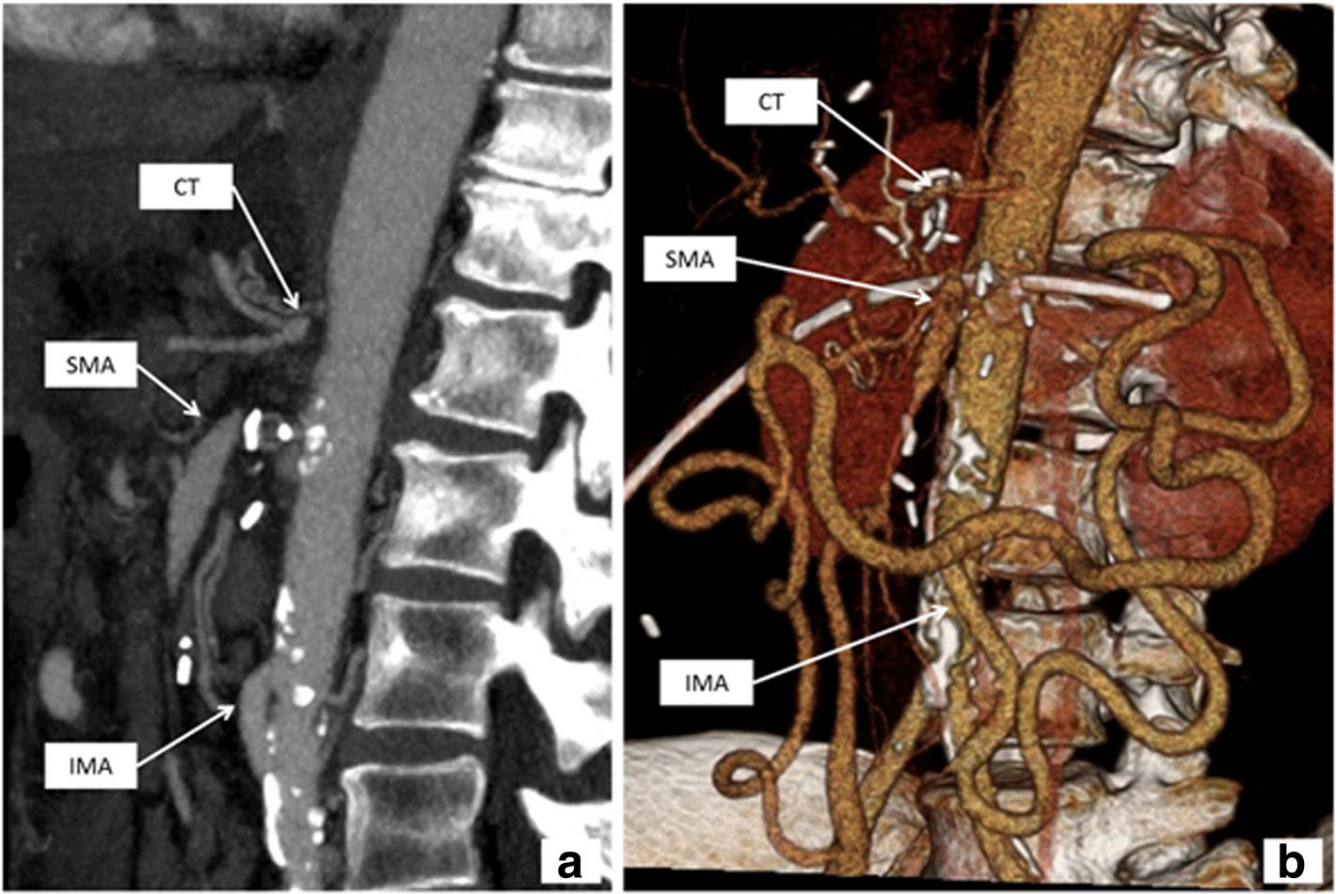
Sagittal reconstruction of a computed tomography scan (a) demonstrates proximal occlusion of celiac trunk (CT) and SMA with poststenotic dilatation of the SMA. Note the hypertrophic IM. Volume rendering (b) shows collateral arteries from IMA to SMA (hypertrophic anastomosis of Riolan)
